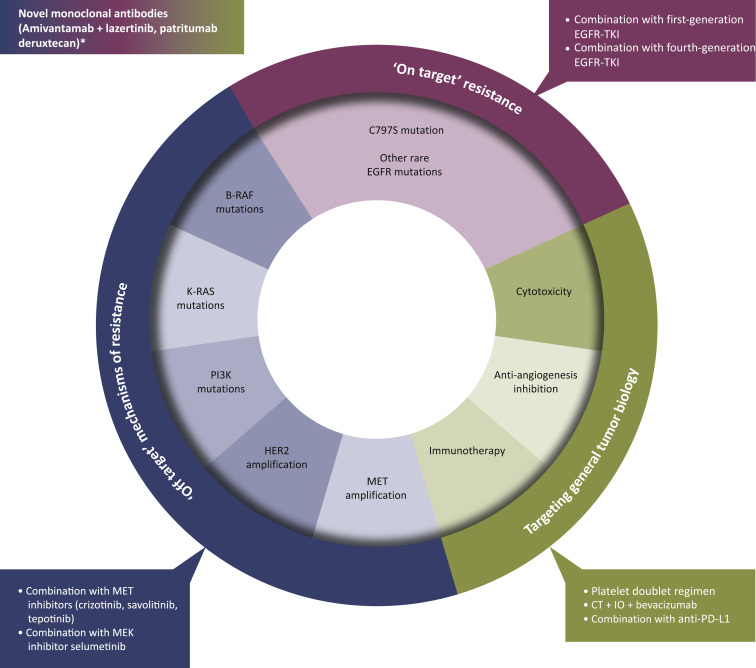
Mechanisms of resistance to osimetinib and potential strategies of treatments to overcome resistance. CT, chemotherapy; IO, immunotherapy. ∗Activity demonstrated across resistance mechanisms.
